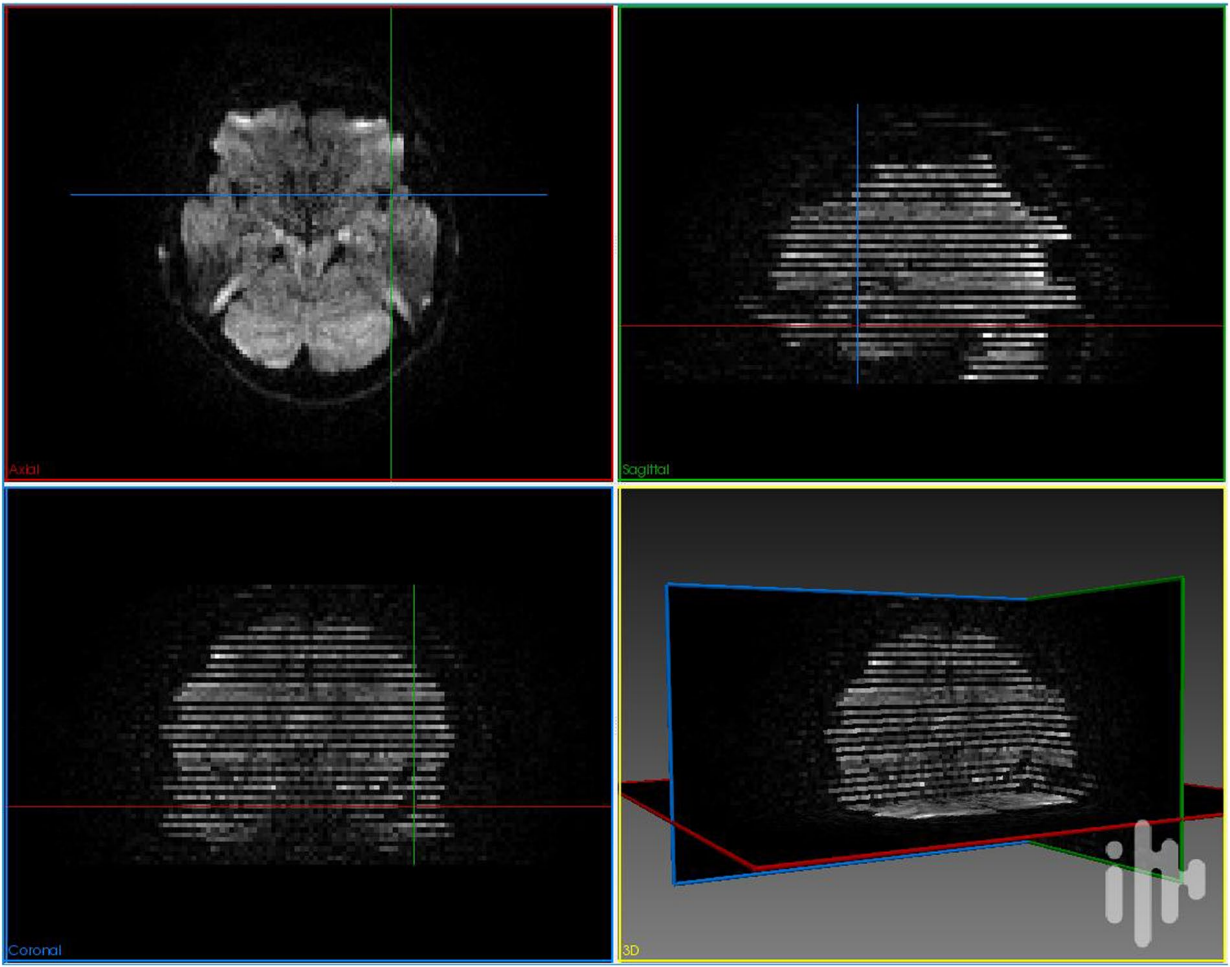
Example of a raw DWI sample that did not pass manual QC because of a slice-drop artifact.
